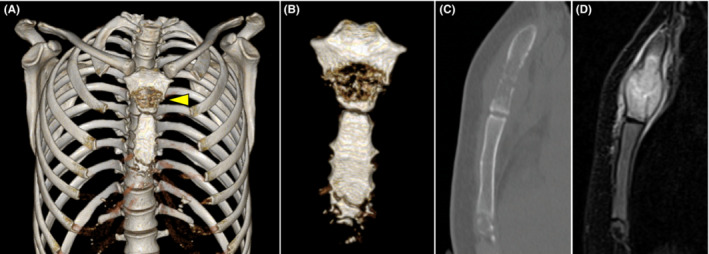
Computed tomography (CT) and magnetic resonance (MR) images of the diseased manubrium/sternum. (A, B) Three‐dimensional reconstruction of the CT image; arrowheads indicate osteolysis of the manubrium. (C) Sagittal CT image; arrowheads indicate osteolysis of the manubrium. (D) Sagittal T2‐weighted MR image; arrowheads indicate the high‐signal intensity in the bone marrow of the manubrium and the extra‐osseous expansion.
